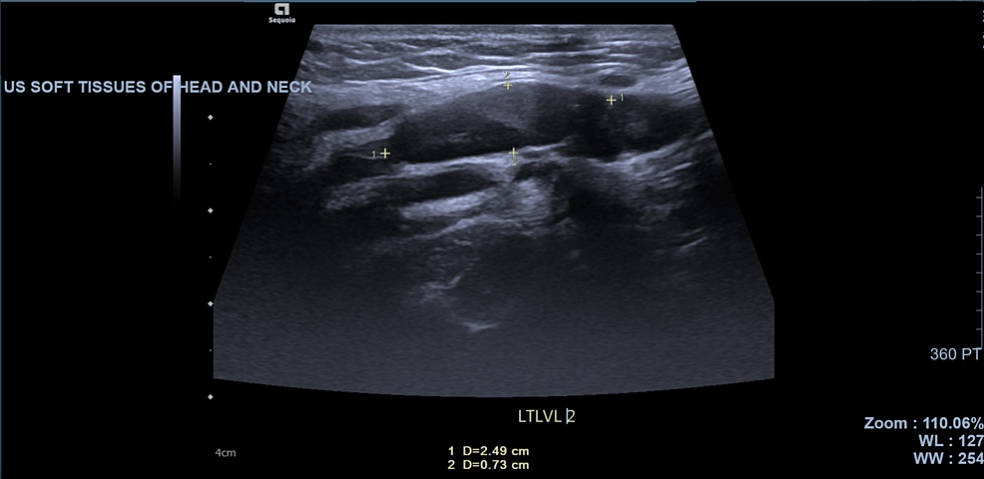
Ultrasonography of the left side of the neck showing left-sided level II lymph node prominence (borders marked with crosses).
